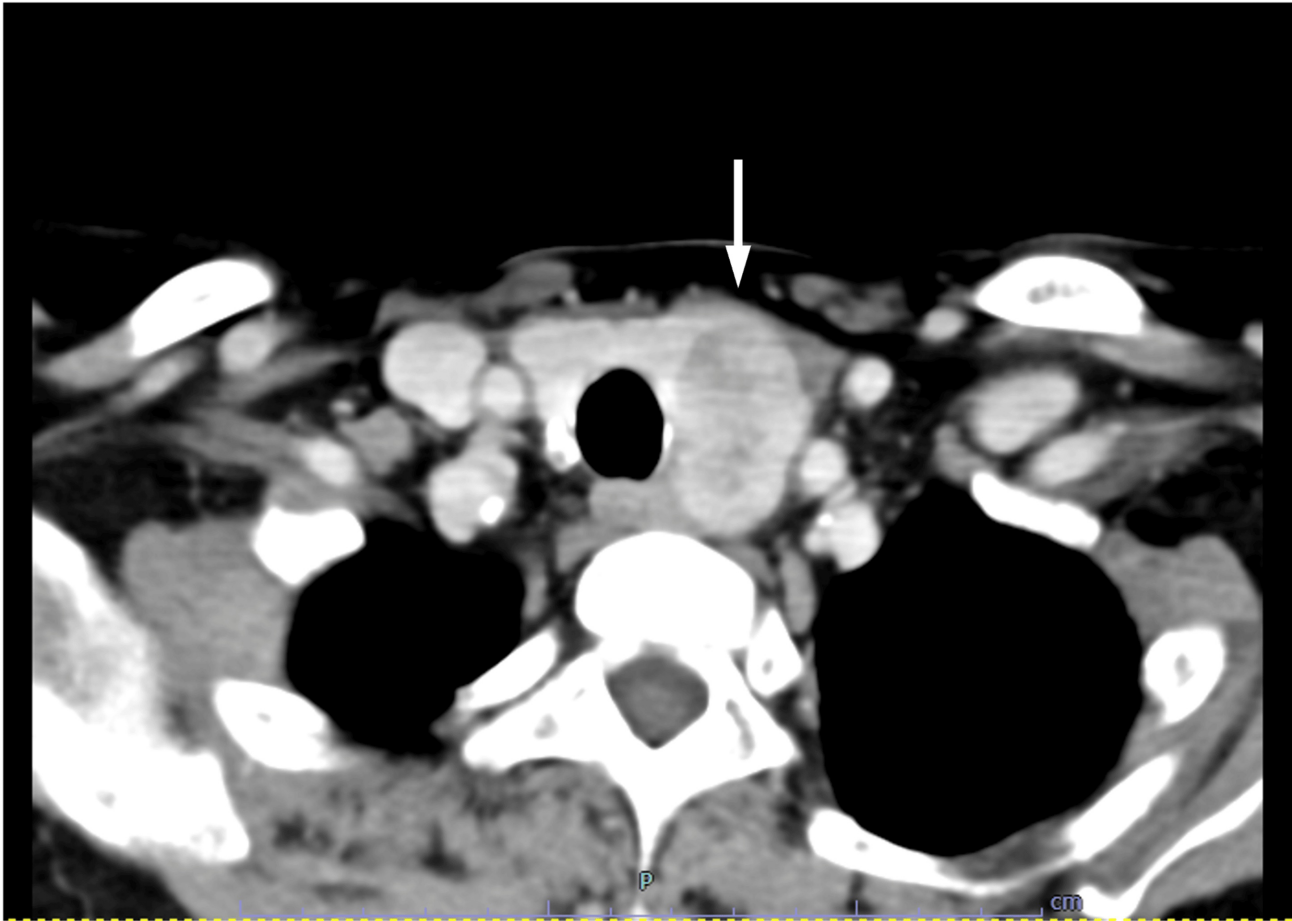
Horizontal slice of neck and chest contrast-enhanced CT scan A lobulated, low-attenuation mass measuring 33 × 21 mm was observed adjacent to the dorsal aspect of the left thyroid lobe (white arrow). Contrast enhancement was noted. No evidence of invasion into the surrounding tissues was observed. CT: computed tomography
